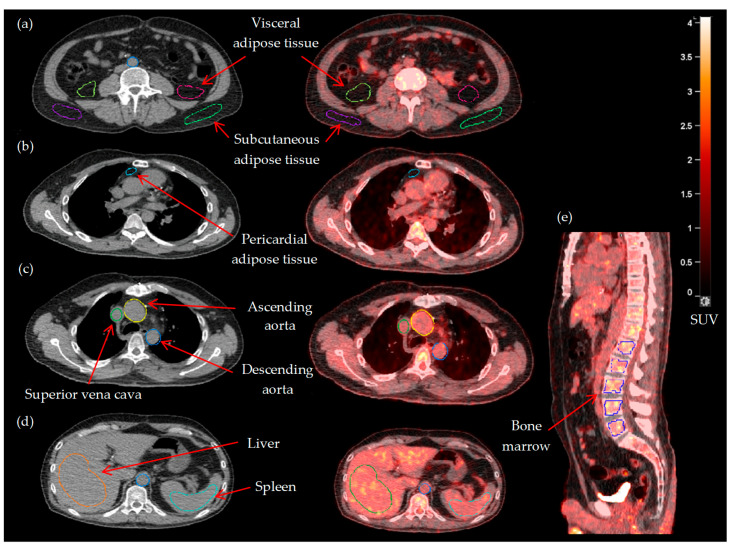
Transverse and coronal images from computed tomography (left) with corresponding positron emission tomography (right) with arrows indicating regions-of-interests (ROIs) drawn in (a) visceral and subcutaneous adipose tissue, (b) pericardial adipose tissue, (c) ascending and descending aorta and superior vena cava, (d) spleen and liver, and (e) bone marrow (lumbar vertebrae 1–5). SUV, standardized uptake value.
